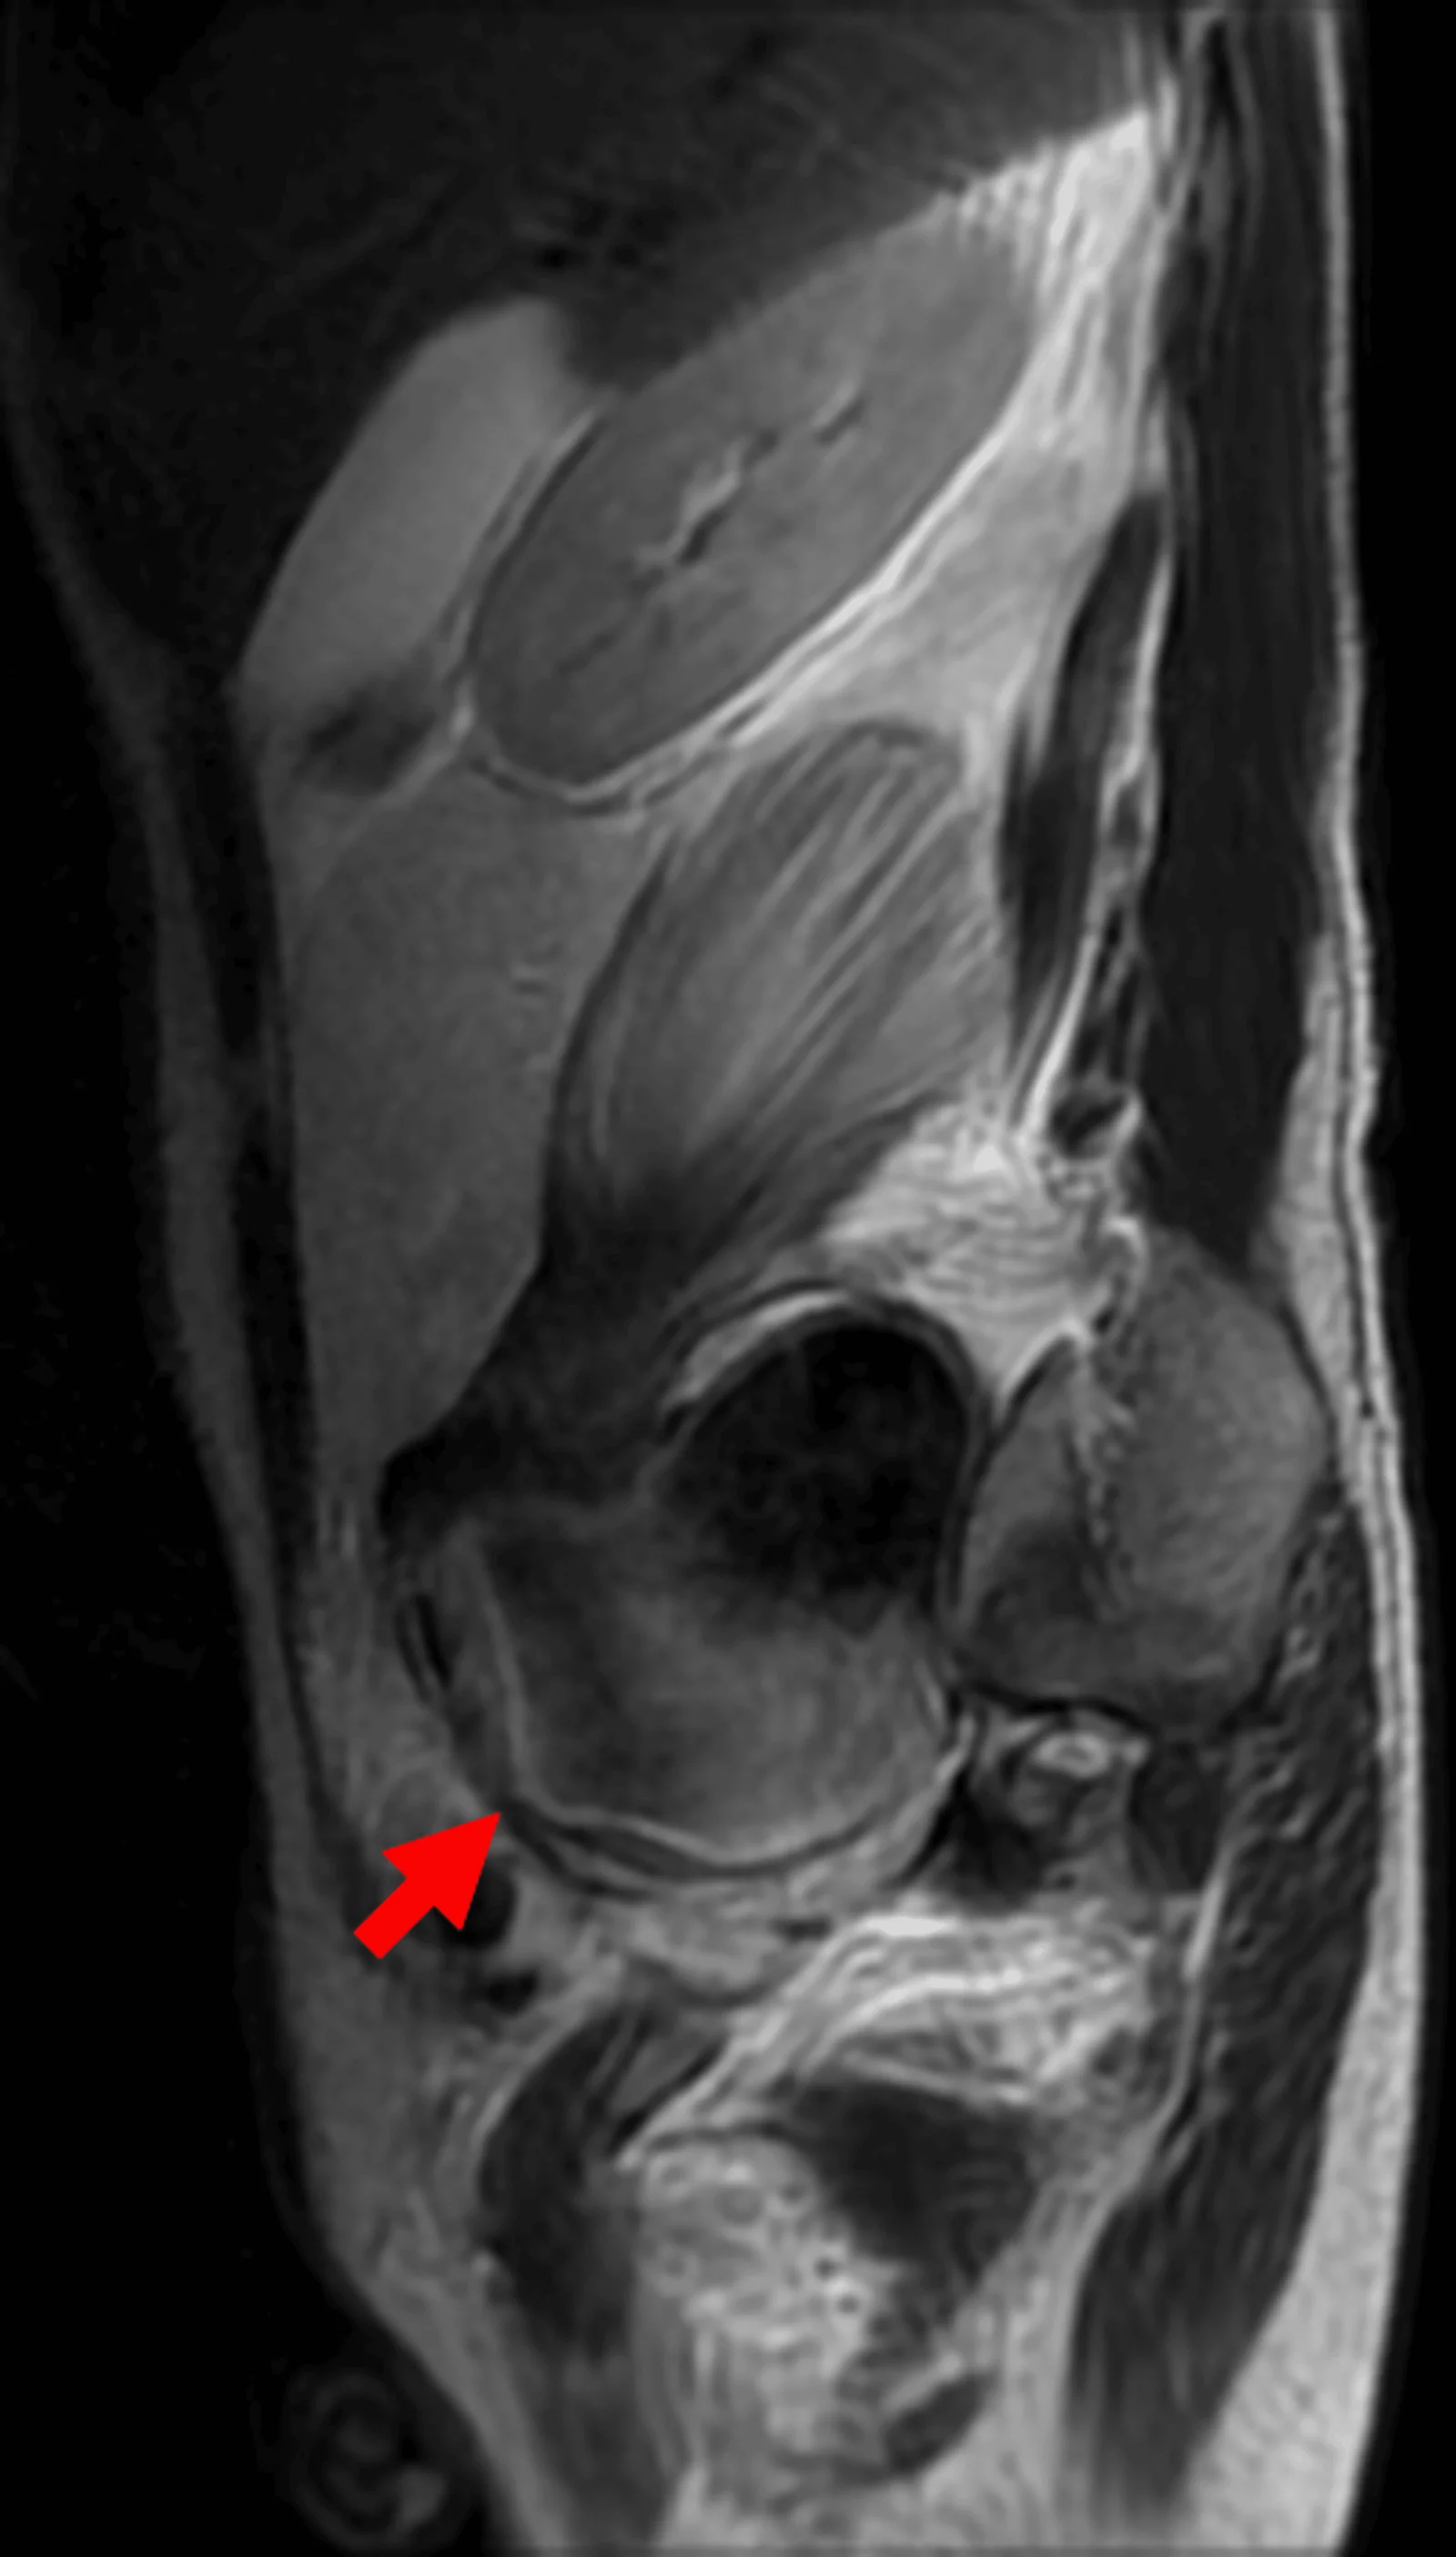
MRI at the referring clinic MRI: magnetic resonance imaging Sagittal MRI obtained during initial evaluation. The red arrow indicates the ruptured internal iliac artery aneurysm
